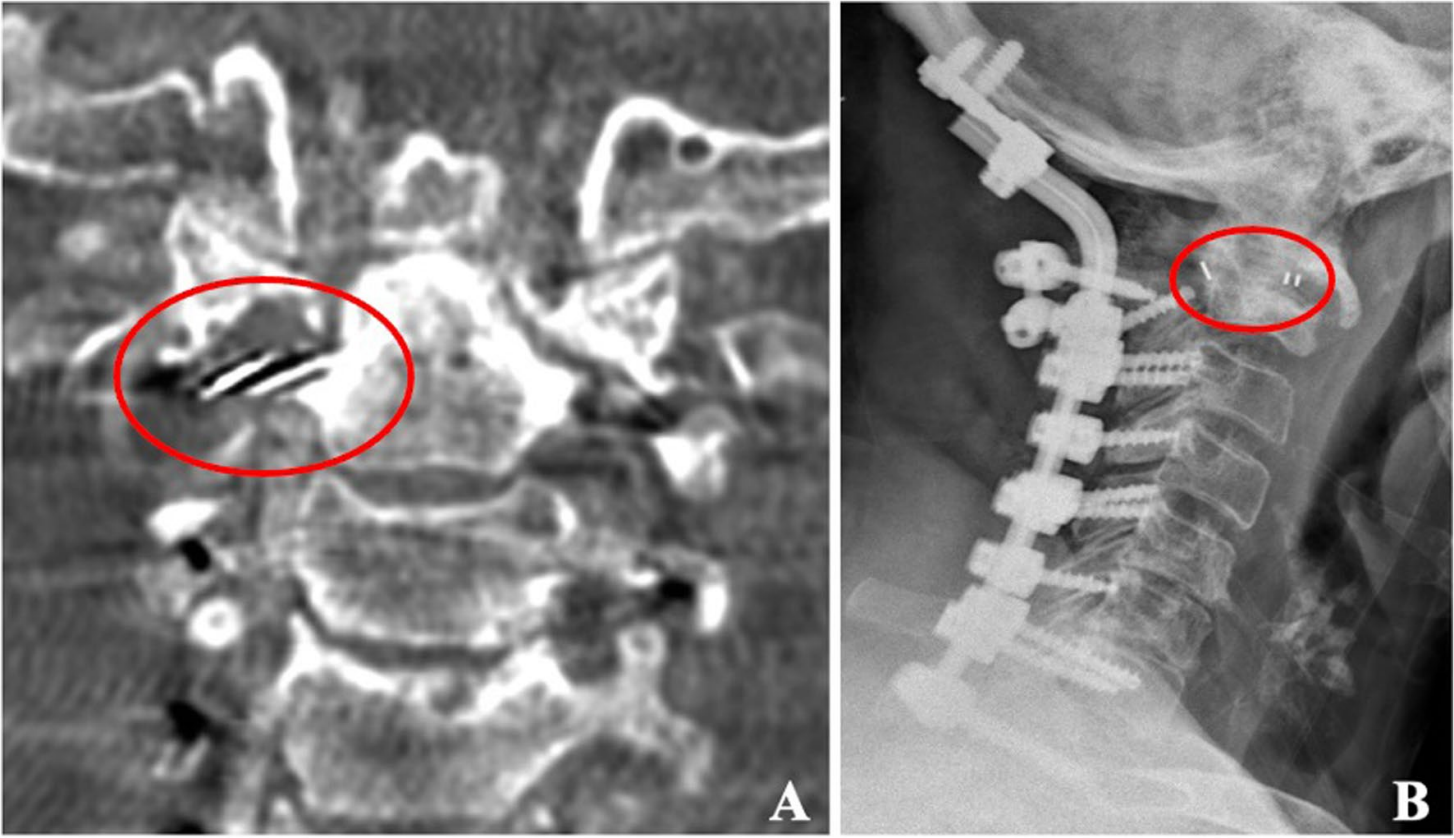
Implantation of unilateral right PEEK cage (red circles) between C1 and C2 articular processes to achieve vertical distraction and correction of basilar invagination via a posterior approach. a CT scan coronal view; b X-ray lateral view
